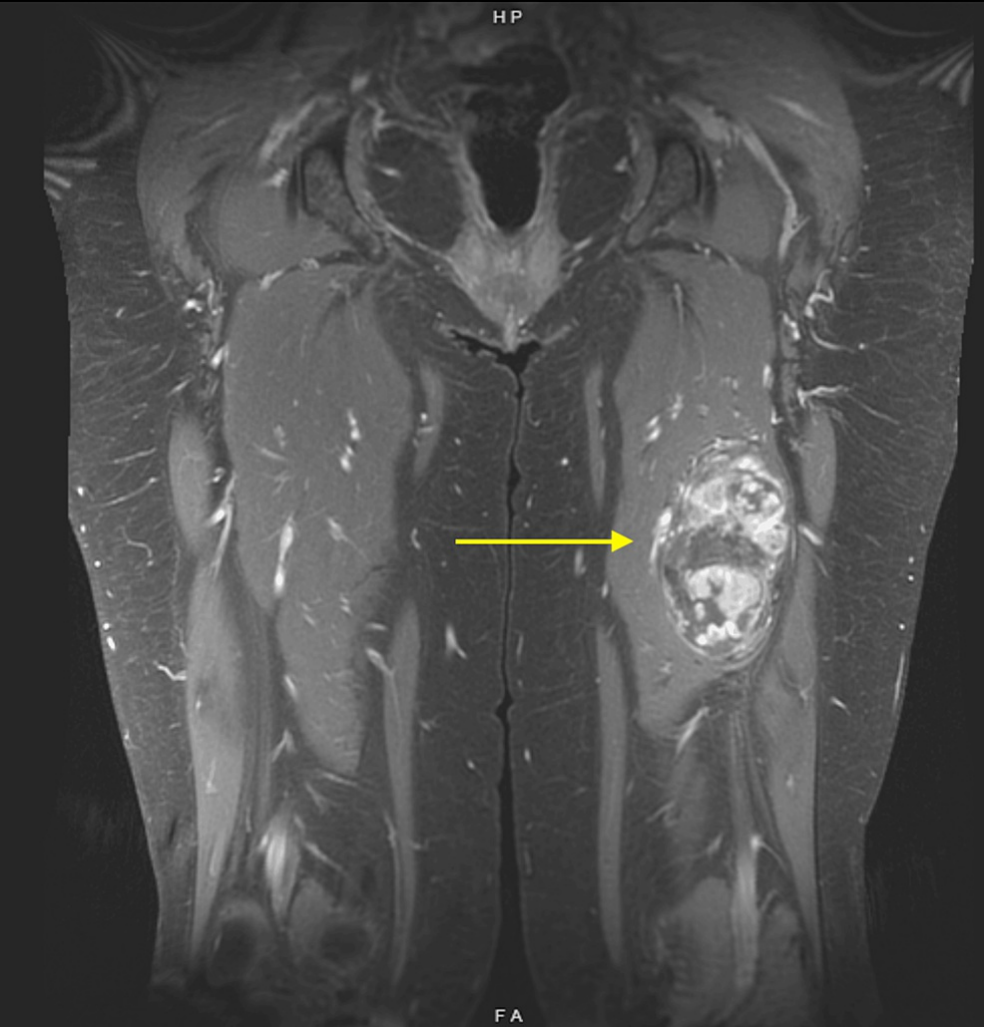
May 2023 MRI of left thigh primary tumor. The yellow arrow points at the tumor.
